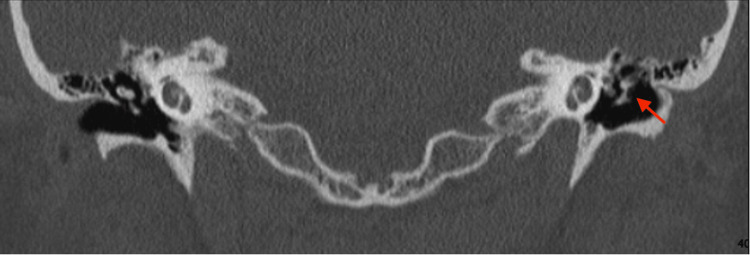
Coronal section of the CT internal auditory meatus This CT scan was performed in 2007 during the initial diagnostic workup. No erosive changes were reported.
